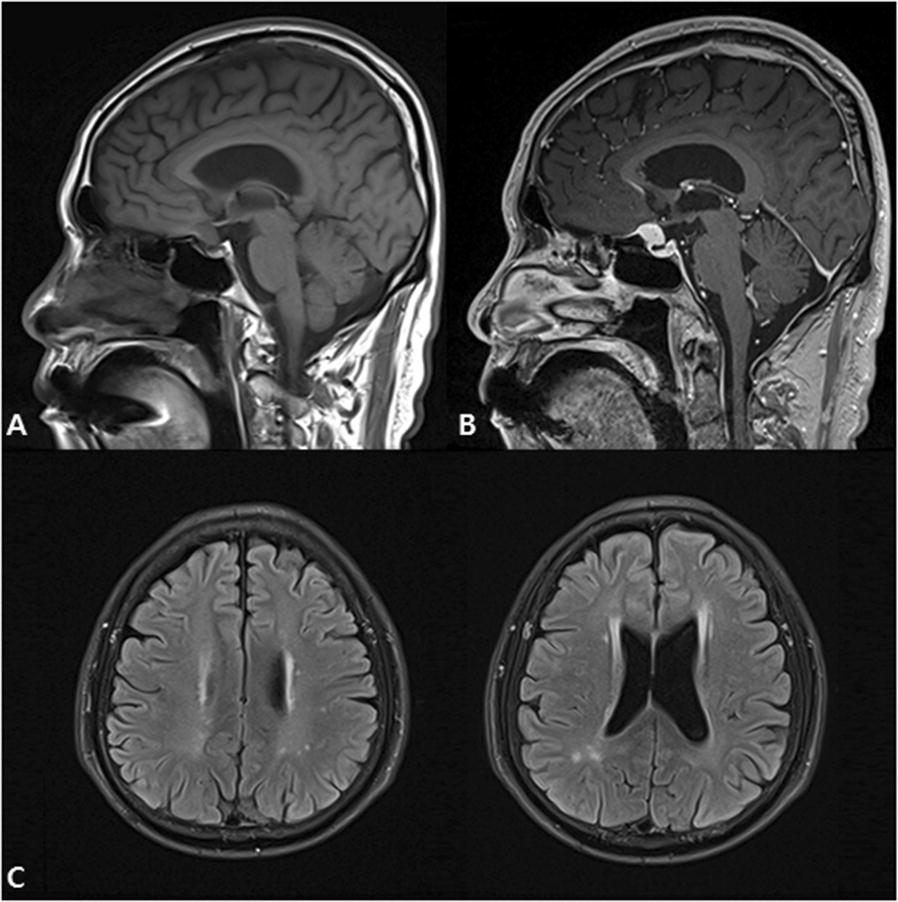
Sagittal T1-weighted (A) pre-contrast and (B) post-contrast MRI showing a tuberculum sellae meningioma measuring 15 × 8 × 13 mm. Axial FLAIR MRI (C) showed multifocal high signal intensities in both the deep and subcortical white matters
